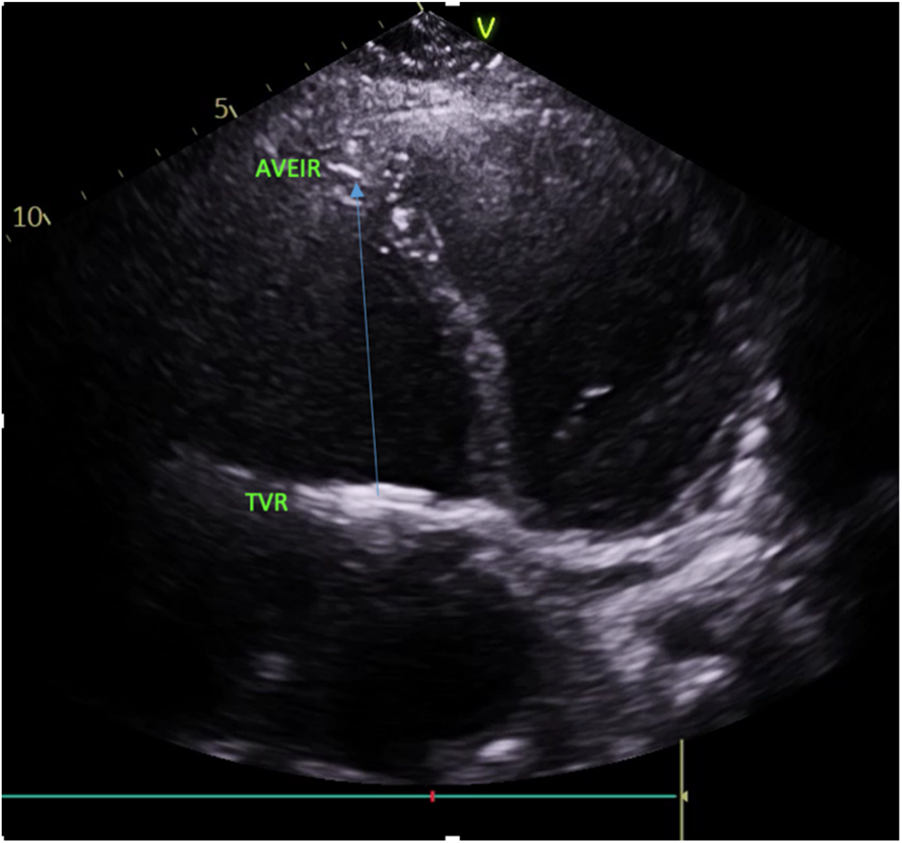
Apical four‐chamber echocardiographic view showing the Abbott AVEIR VR leadless pacemaker in the right ventricular apex, with clear visualization of its distance from the metallic tricuspid valve replacement (TVR) and preserved right ventricular function.
